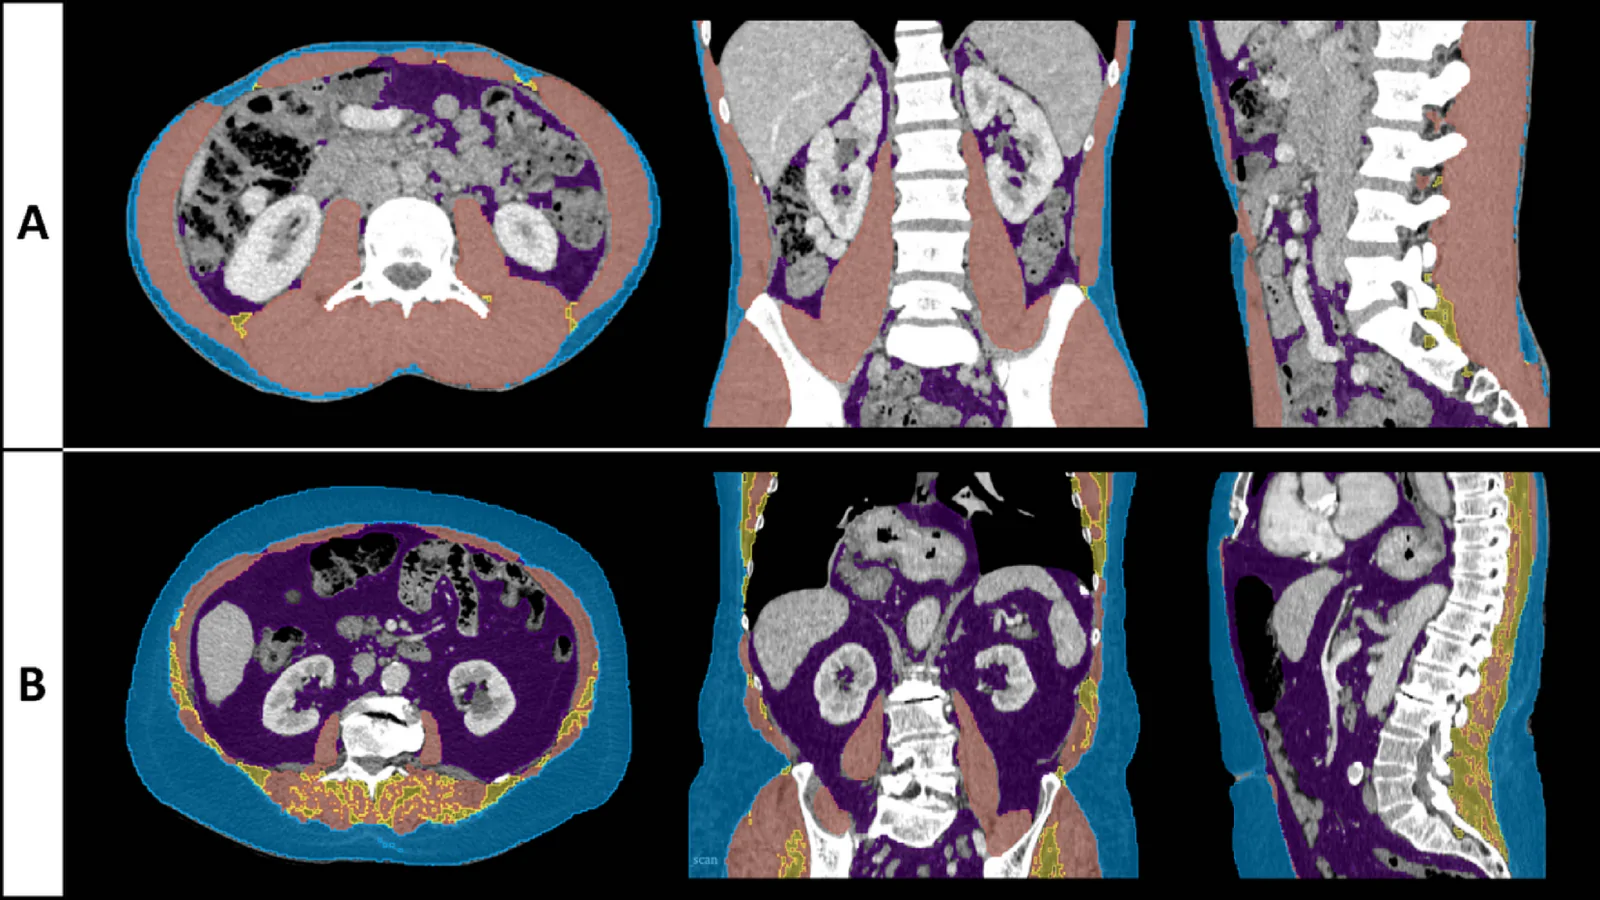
Representative examples of body composition extremes on CT imaging: (A) a patient with high skeletal muscle mass and low adipose tissue, and (B) a patient with low skeletal muscle mass and high adipose tissue.
